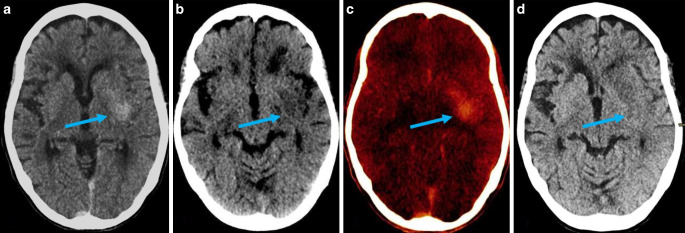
A hyperdensity is seen in the left basal ganglia (*blue arrow*) on a standard non-contrast mixed image from TwinSpiral dual-energy CT image in an acute ischemic stroke patient after endovascular thrombectomy (a). No hyperdensity is seen in the same location on the VNC image (b). Hyperdensity is seen on the iodine map (c). Follow-up non-contrast CT (d) showing no hyperdensity at the corresponding location (*blue arrow*), thus indicating the contrast extravasation resorbed in follow-up imaging
